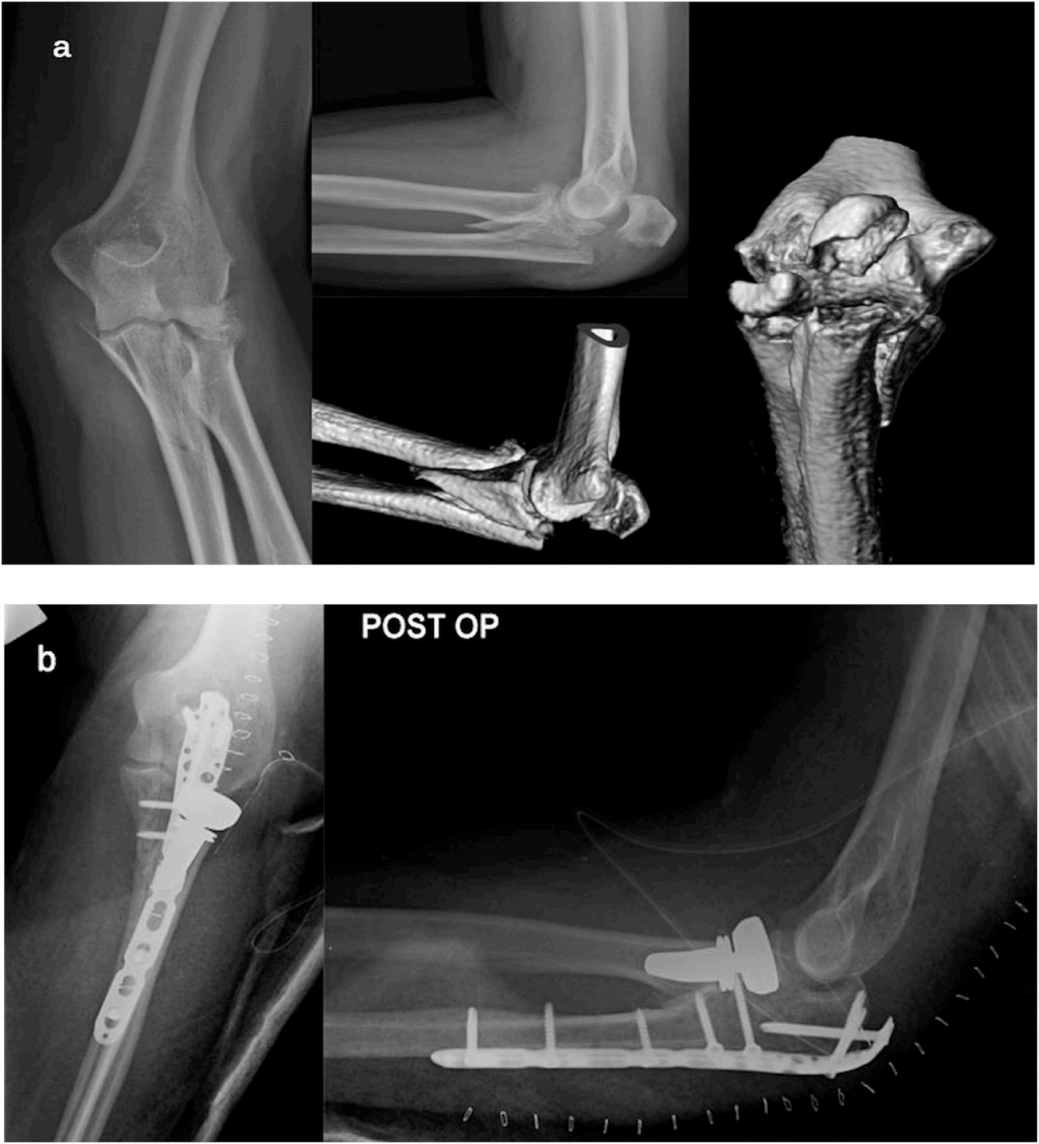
a) Pre-operative radiography and CT scan: fracture of the proximal ulna and coronoid fracture (large fragment); comminuted fracture of radial head in a 66-year old woman. b) Post-operative radiography: global posterior approach and subcutaneous Kocher approach – Free lag screws for coronoid fracture. Pre-contoured locking compression plate and radial head prosthesis.
